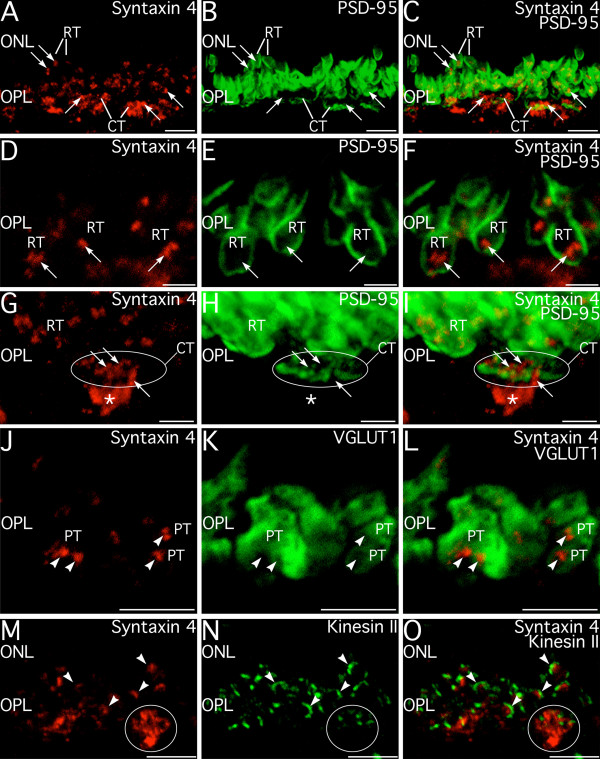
Syntaxin 4 labeling in the outer plexiform layer (OPL) is associated with processes post-synaptic to the photoreceptor terminals, not the terminals themselves. A–C: Double labeling for syntaxin 4 and the photoreceptor terminal marker PSD-95 show that syntaxin 4-positive puncta (arrows) are spatially associated with rod terminals (RT) in the distal OPL and with cone terminals (CT) in the proximal OPL. Images shown from a projection of 27 optical sections with total thickness of 3.79 μm. D–F: At high magnification, syntaxin 4-positive puncta in the distal OPL appear as singlets or doublets (arrows) enveloped by the terminals of rods (RT) labeled for PSD-95. Image shown is a projection of 22 optical sections with total thickness of 3.2 μm. G–I: In the proximal OPL, syntaxin 4 labeled structures (arrows) are associated with cone terminals (CT). Several syntaxin-4 positive puncta are enveloped by the cone terminal. In addition, syntaxin 4 labeling is localized to a cluster of processes in the OPL just proximal to the cone terminal (*) that can be seen to connect to the puncta within the cone terminal. Numerous rod terminals (RT) and associated syntaxin 4 puncta are visible distal to the cone terminal. Image shown is a projection of 34 optical sections with total thickness of 7.09 μm. J–L: Syntaxin 4-labeled structures (arrowheads) in the OPL do not colocalize with synaptic vesicles labeled for VGLUT1 in photoreceptor terminals (PT). Images shown from a projection of 13 optical sections with total thickness of 1.75 μm. M–O: Synaptic ribbons in rod and cone terminals do not label for syntaxin 4. However, syntaxin 4-positive puncta in the OPL are spatially associated with synaptic ribbons and often can be seen to follow the contours of the ribbons (arrowheads) labeled for kinesin II in rod and cone terminals. Circle indicates location of a cone terminal. Images shown from a projection of 12 optical sections with total thickness of 1.60 μm. ONL, outer nuclear layer. Scale bars = 5 μm for A–C, and J–O; 2 μm for D–I.
